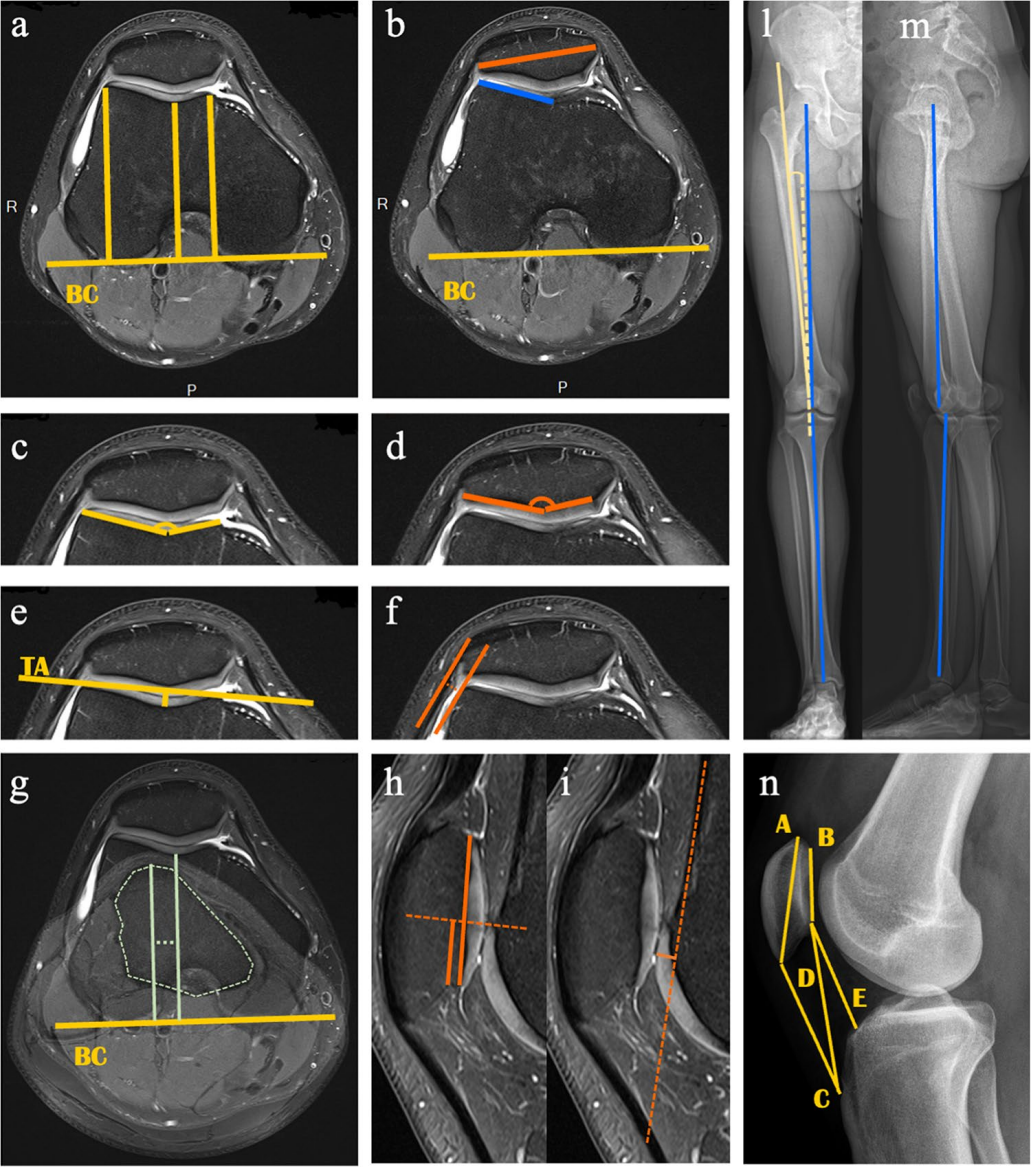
Images of performed measurements on 3 T MRI, EOS, lateral X-rays. A detailed description can be found in Table 2. Bicondylar line (BC line) (a, b, g); anteroposterior femoral distance (maximal medial/lateral condyles; minimal trochlear groove) (a); lateral trochlear inclination (blue) and patellar width and inclination angle (orange) (b); trochlear medial and lateral facets length and asymmetry of the facet length and sulcus angle (c); patellar medial and lateral facet length, facet angle and asymmetry (d); trochlear axis (TA line) and trochlear groove depth (e); lateral patellar displacement (f); TT-TG (g); patellar height, patellar-trochlear overlap and index (h); ventral trochlear prominence (i); femoral-tibial angles (l, m); Q angle (l); Insall–Salvati ratio, modified Insall–Salvati ratio, Caton–Deschamps ratio (n)
